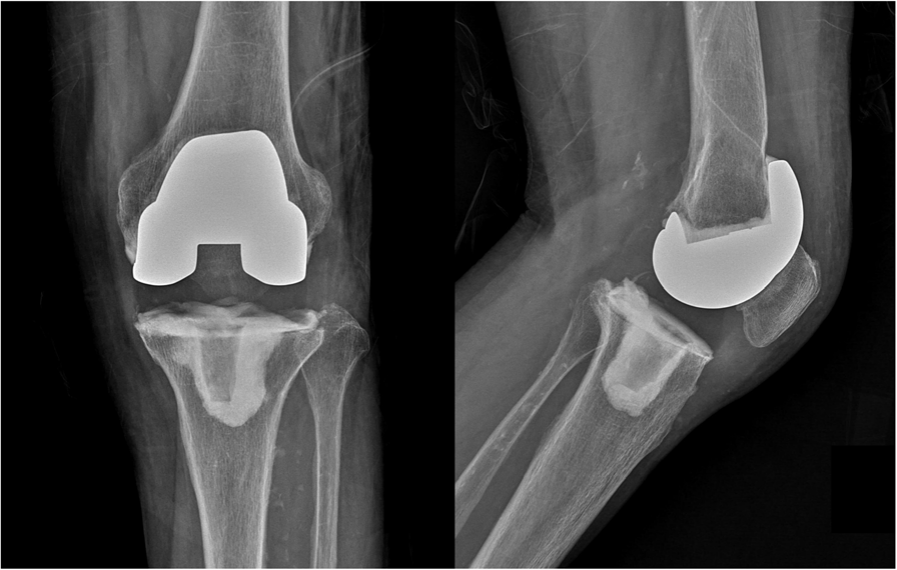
Postoperative plain radiograph of resection arthroplasty. The infected prosthesis was removed, and antibiotic-mixed cement was placed on the articular side of the femur and tibia
